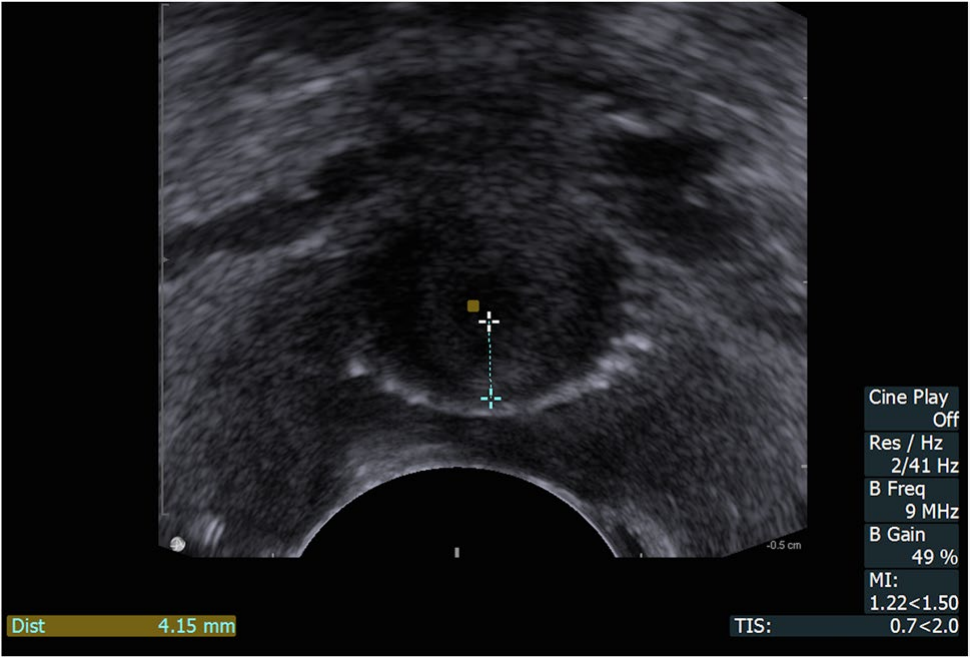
Ultrasonographic tape visualisation in a transverse scan: Dist: distance between the tape and the urethral lumen (T_UL_)
